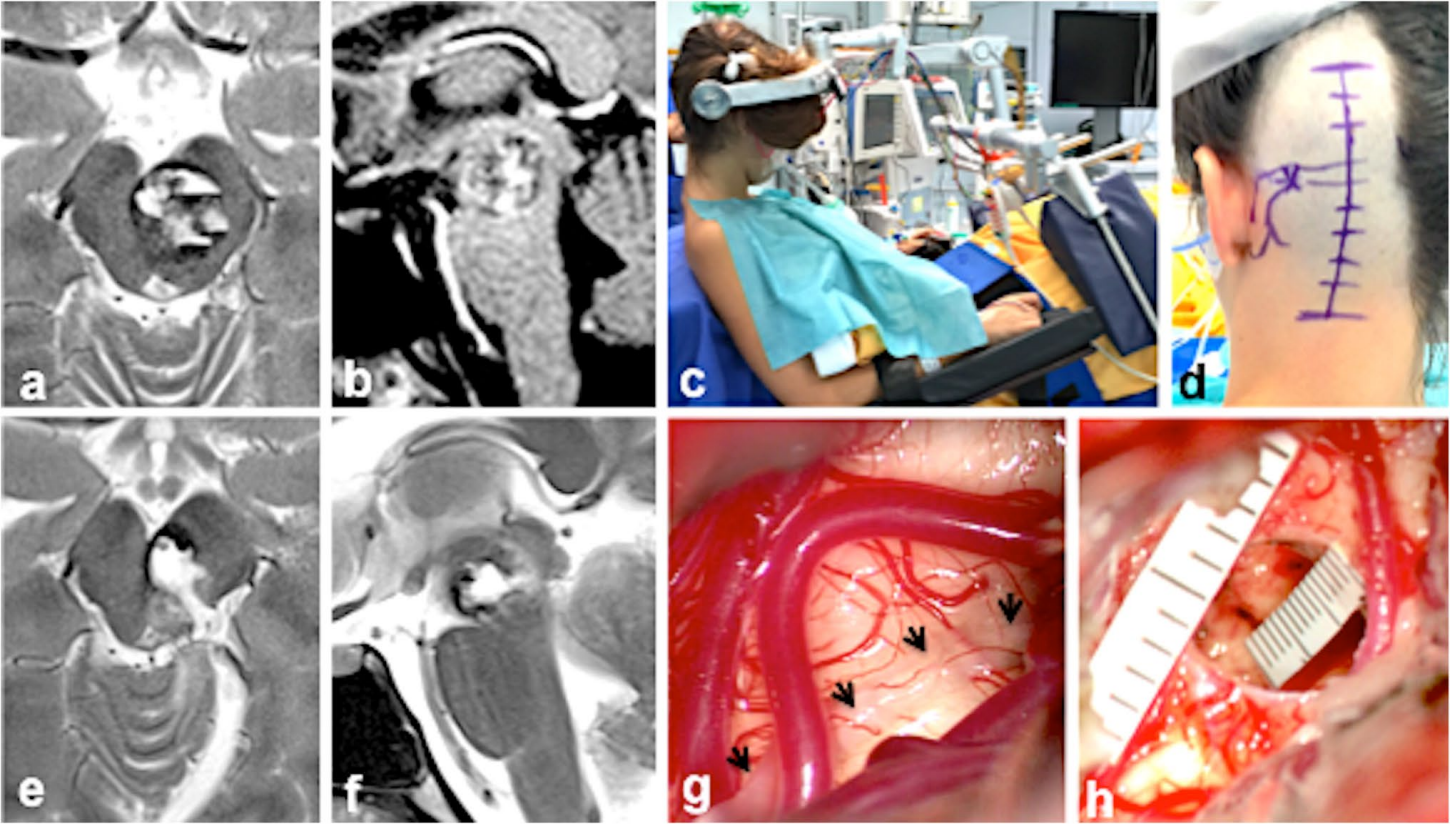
This 19-year-old female presented with a history of two repeated hemorrhages from an intra-axial MCM. Her symptoms consisted of headache, diplopia, and slight motor weakness of her right-sided body. Preoperative axial (a) and sagittal (b) MRI showed a 20-mm-sized type *nl* MCM in the tegmentum region. She underwent surgery in the semi-sitting position (c) via a lateral supracerebellar transtentorial approach; a left-sided lateral occipital/suboccipital longitudinal incision was marked on skin (d). Postoperative axial (e) and sagittal (f) MRI confirmed total lesionectomy. At surgical exposure, the lateral surface of the tegmentum appeared normal; the arrowheads indicate the course of the trochlear nerve (g). The intraoperative photograph taken after complete MCM removal shows the empty resection cavity with two millimetre  scales (h). Postoperatively, a left-sided partial oculomotor paresis was present that gradually improved over the following weeks up to normal function. No other neurological deficits were noted
